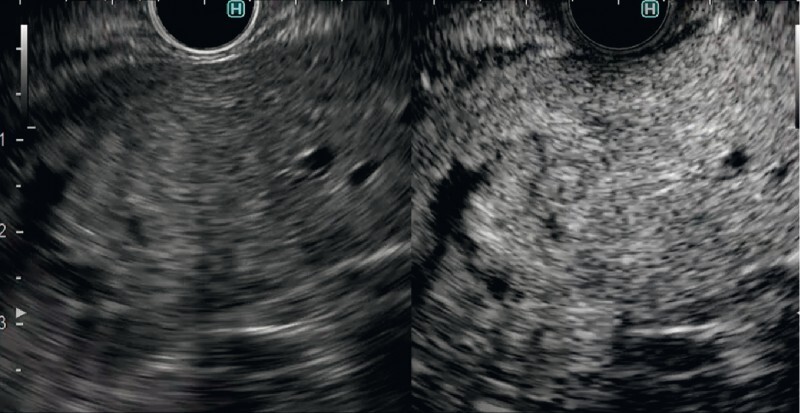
Contrast-enhanced harmonic endoscopic ultrasound image showing homogeneous enhancement of the tumor that makes evaluation of tumor hemodynamics impossible.
